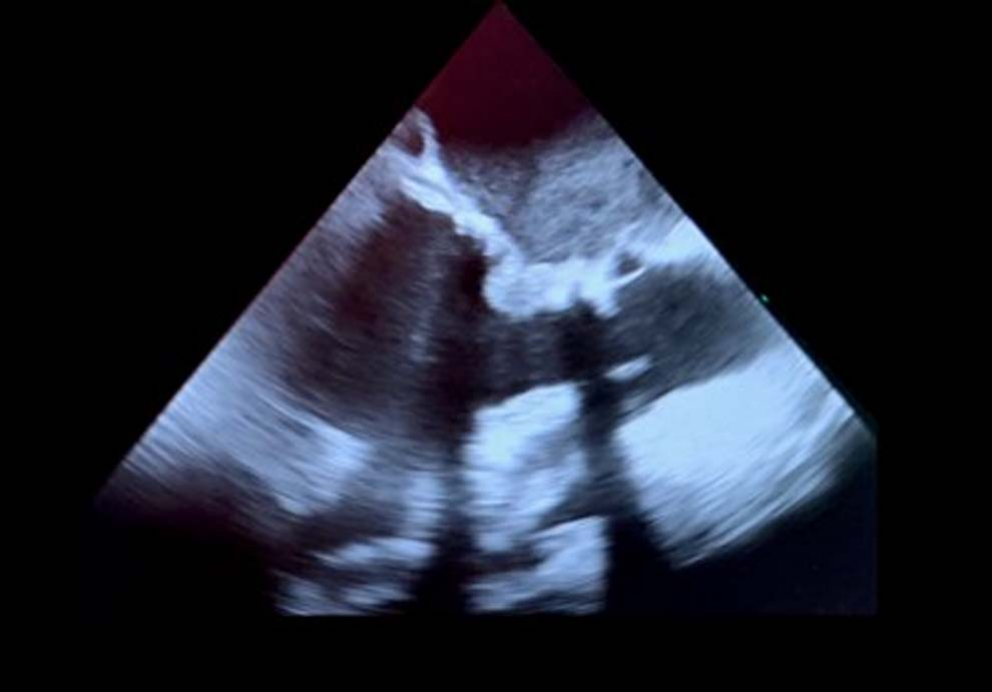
Preoperative ultrasound transesophageal echo-cardiography shows the thickened mitral valve obstructing cardiac vascular flow.
